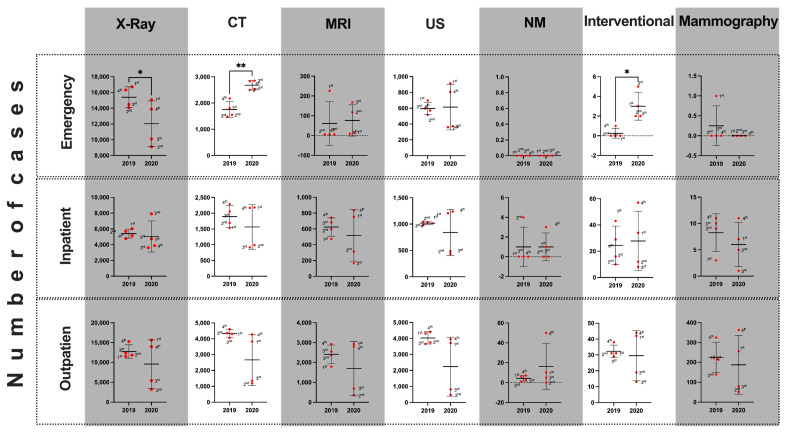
A comparison of the total number of cases performed by different imaging modalities (columns) at different departments (rows) between 2019 and 2020. CT= Computed Tomography, MRI= Magnetic Resonance Imaging, US= Ultrasound, NM= Nuclear Medicine.
